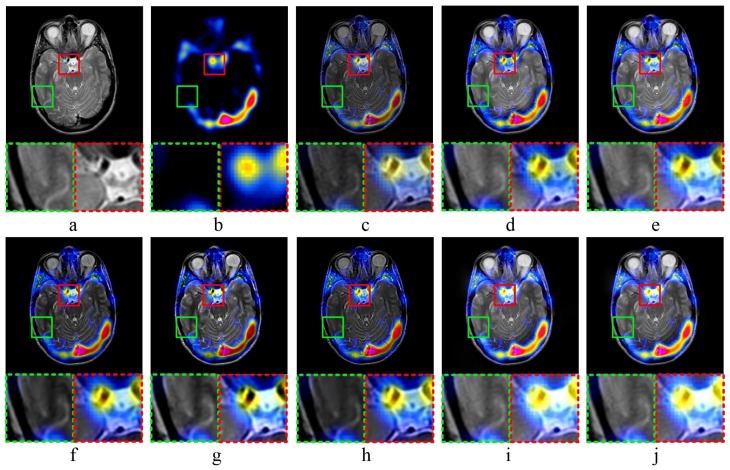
MR-SPECT image fusion Experiment 1: (a,b) source images; and (c–j) the fused image obtained by MST-SR, NSCT-PC, NSST-PCNN, ASR, CT, KIM, CNN-LIU, and the proposed method, respectively. Two partially enlarged images marked in green and red dashed frames correspond to the regions surrounded by green and red frames in the fused image.
